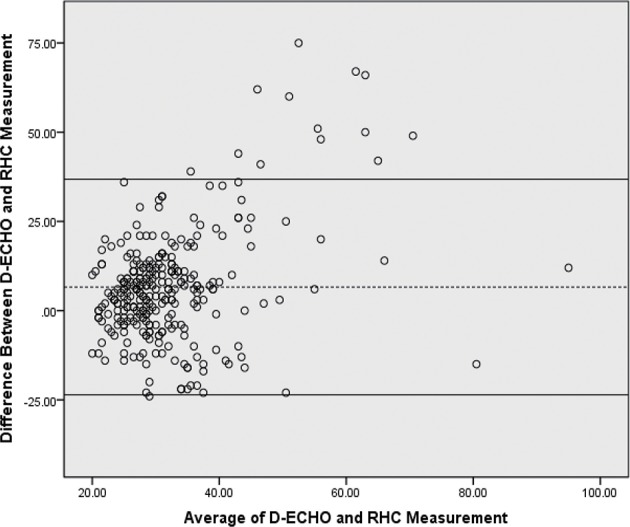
Bland-Altman plot of sPAP estimated by Doppler echocardiographic and RHC. The bias was 6.6 mmHg and the 95% limits of agreement were 23.6–36.8 mmHg. Dotted line, bias; solidline curve, upper and lower limits of agreement. D-ECHO, Doppler echocardiography; sPAP, pulmonary artery systolic pressure; RHC, right-heart catheterization.
